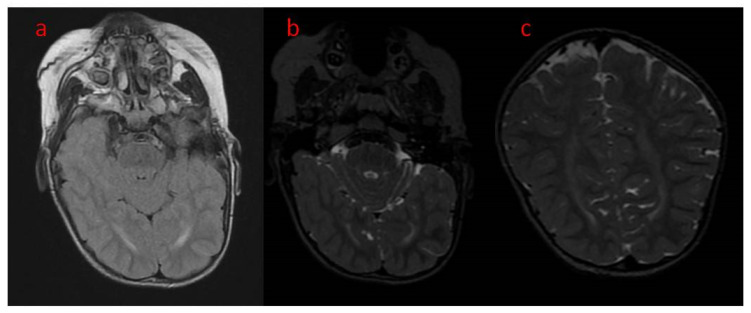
(a–c) Brain MRI, axial (a) T2 FLAIR (b) T2, coronal (T2) unfinished myelinisation of white matter surrounding occipital horns.
